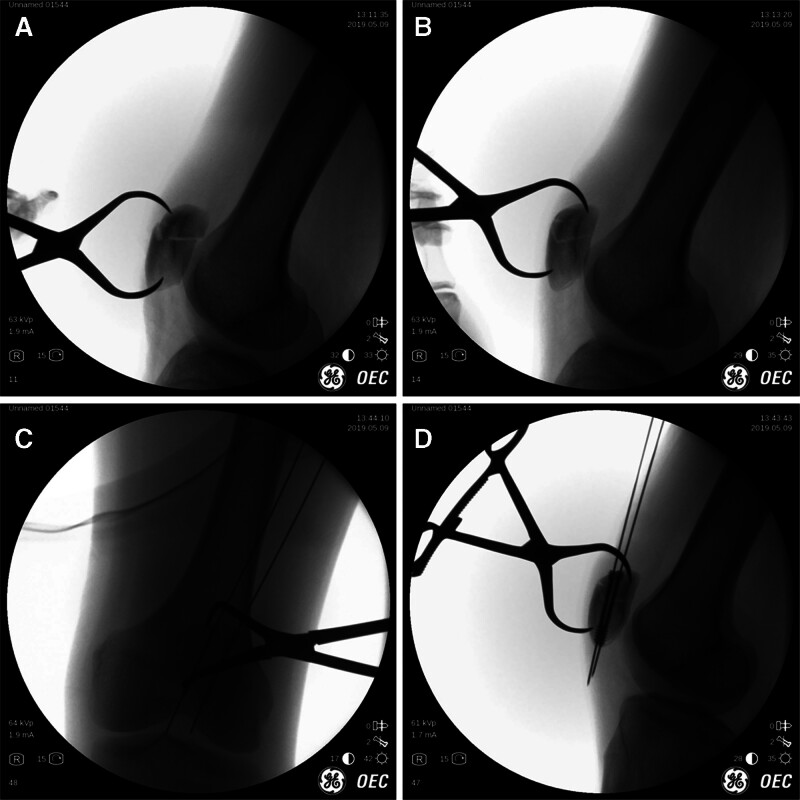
Percutaneous reduction and cannulated screw fixation assessed with C-arm. (A) Application of a reduction clamp across fragments percutaneously, (B) satisfactory reduction achieved with the clamp, (C) anteroposterior, and (D) lateral X-ray films of cannulated screw fixation through the guide wires.
